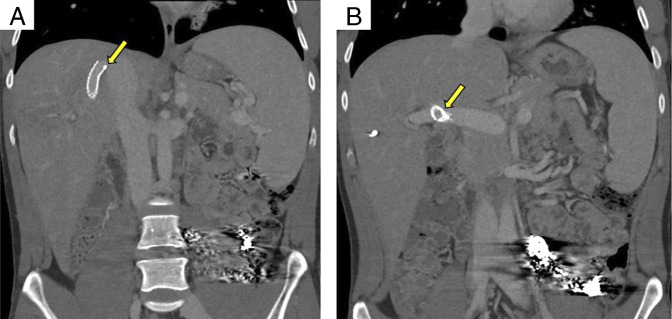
(A and B) Coronal section of abdominal computed tomography showing transjugular intrahepatic portosystemic shunt stent between right portal vein and right hepatic vein.
